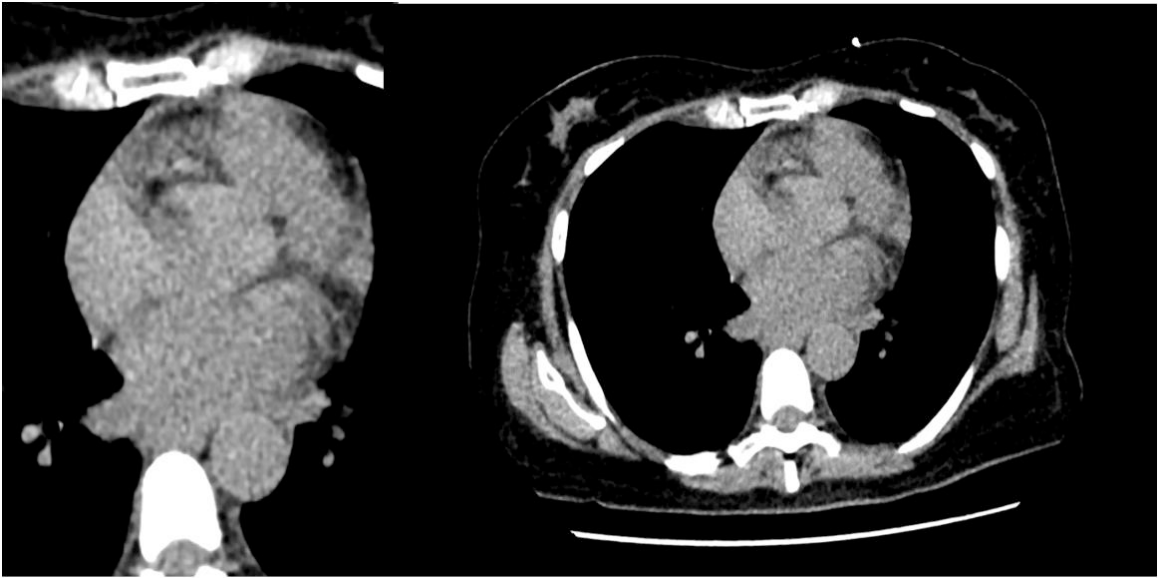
Initial simple chest computed tomography. There are no demonstrable alterations in the mediastinum, changes in the peri-oesophageal fat, pericardial effusion, or pneumopericardium.
